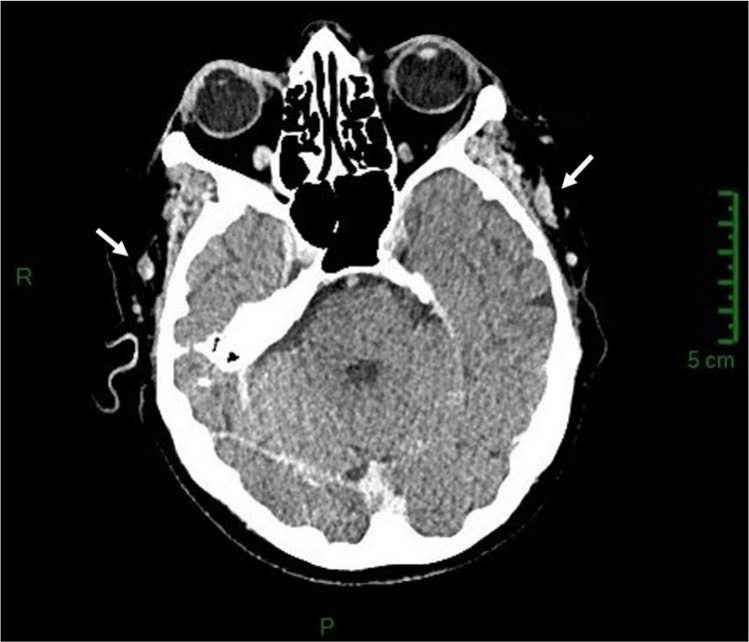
Axial section of the brain computed tomography scan showing thrombosis of the two superficial temporal veins (arrows).
